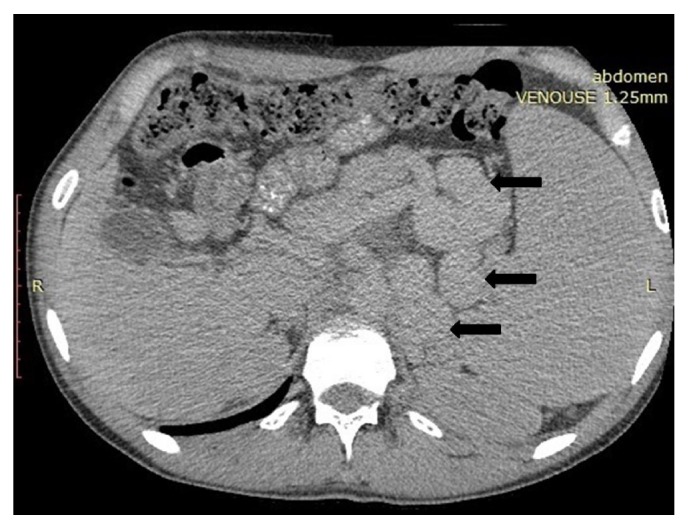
Axial postcontrast CT shows voluminous splenorenal collaterals (arrows).
